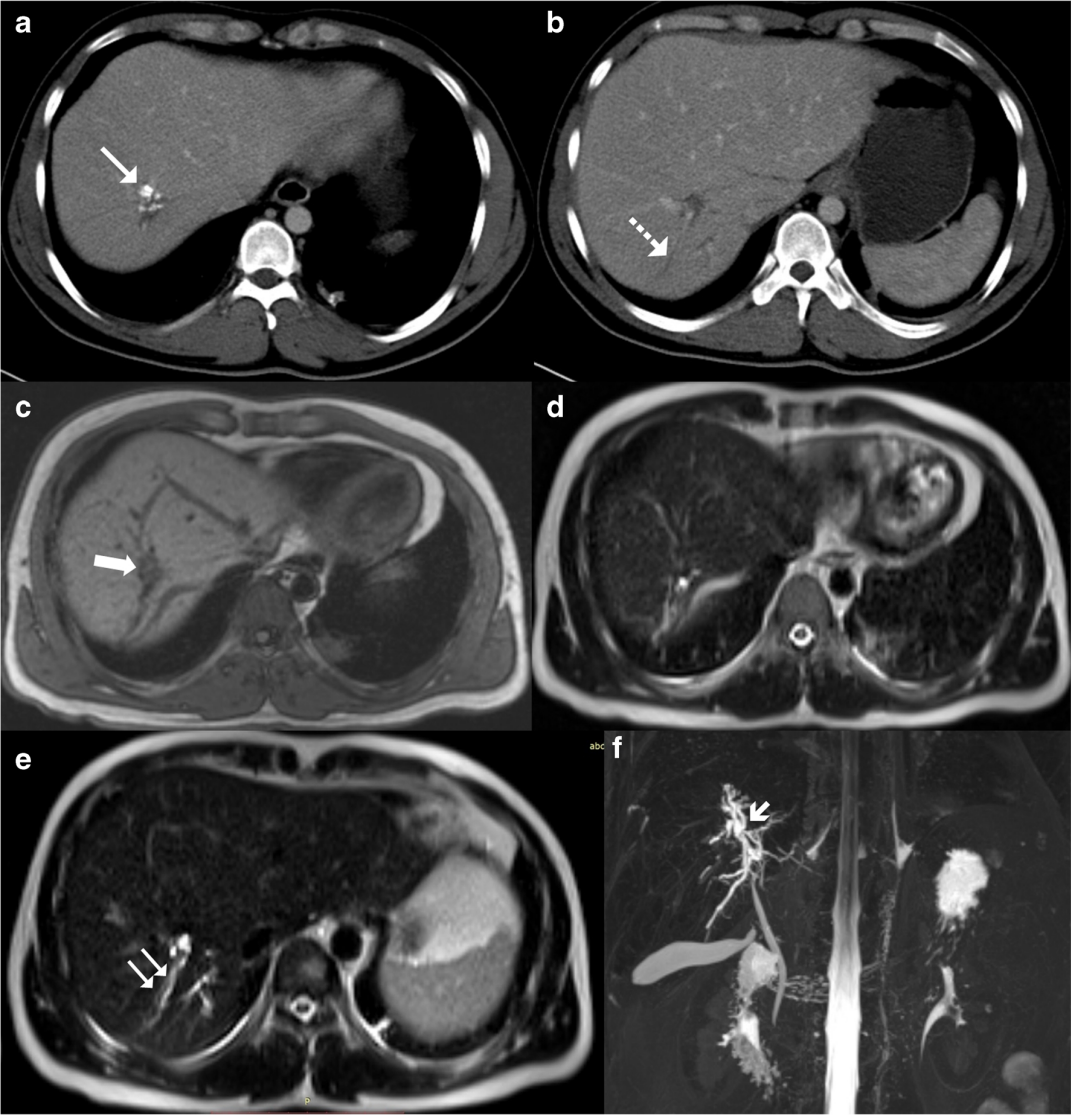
A 35-year-old man previously treated for pulmonary tuberculosis now presenting with elevated alkaline phosphatase. a, b Axial contrast-enhanced CT reveals chunky calcified nodular lesion in the segment VII of right lobe (*arrow*) with adjacent biliary radical dilatation (*dotted arrow*). b, c Axial T1- and T2-weighted MRI shows the corresponding lesion to be hypointense on T1 (*thick arrow*) and isointense to liver parenchyma on T2-weighted sequence. e, f Axial T2-weighted MRI reveals focal biliary radical dilatation (*paired arrows*), which is very well depicted on the MRCP image (*short arrow*)
